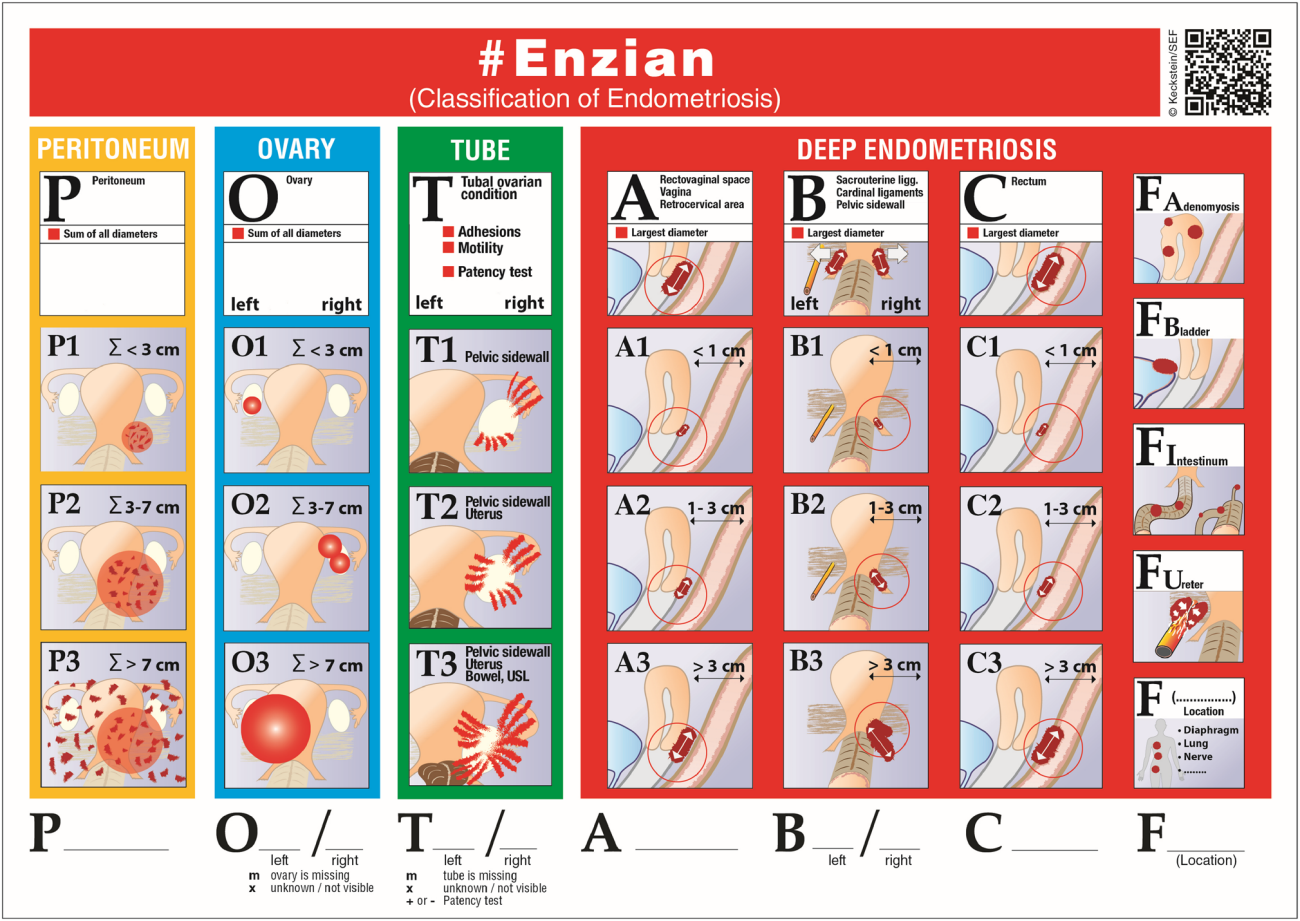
#Enzian classification for the comprehensive description and classification of endometriosis. The individual affected compartments are classified according to the localization and size of the findings using a code. The compartments are marked with capital letters and in the case of paired organs or structures (ovary, tube, USL and ureter); the sides are also shown separately behind the respective letters. The lesions are classified with a code that takes into account both the location and the size of the different findings. The results of soft markers (sliding signs) and tube perturbation (e.g., with HyCoSy) are also shown. The classification can be used for both non-invasive (TVS = (u), MRI = (m)) and invasive ((s) = surgery) diagnostics
